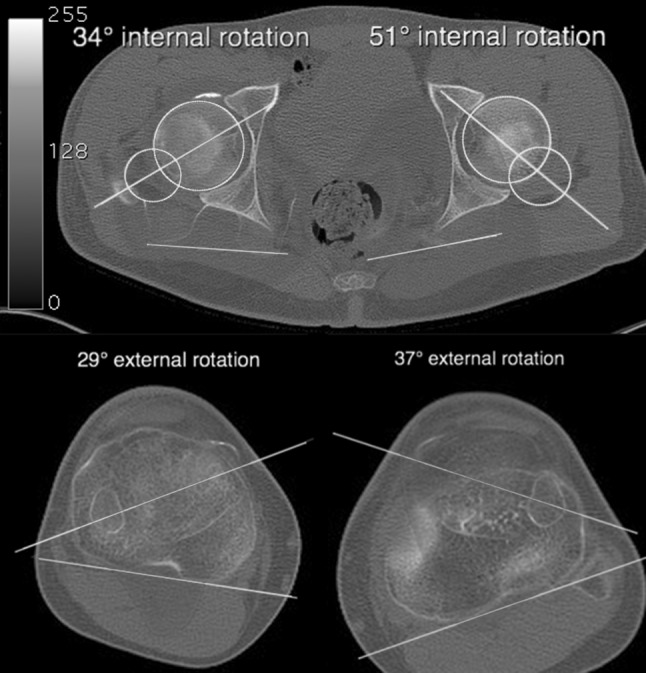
Preoperative CT scan. Analyses of the rotational deformity of the femur and the tibia using Osirix MD (Switzerland). Method of measurement according to Waidelich [10]
